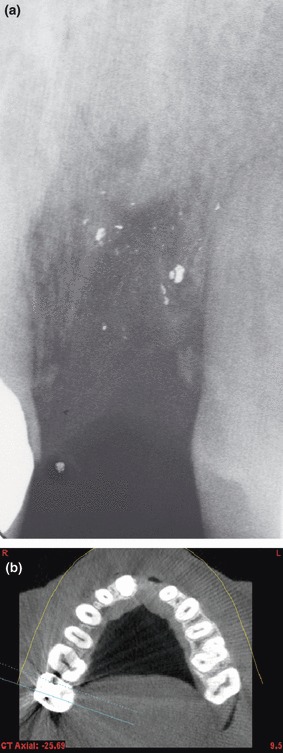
(a) This patient suffered trauma to the 21 which was subsequently treated with orthograde endodontics followed by apical surgery. Because of persistent infection, the tooth was extracted. (b) CBCT examination revealed an obvious bony defect which was not amenable to implant placement. The feasibility of bone graft placement was also difficult to predict because of the lack of bone present to receive donor tissue.
